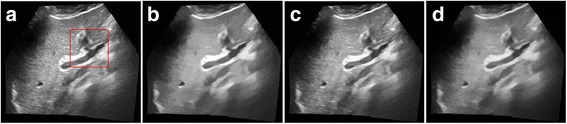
Results obtained with the compared filters applied to the 3D ultrasound image. a The original image. b-d Outputs of the compared filters, b BNLTV, c SAR-BM3D, d The proposed method. BNLTV = Bayesian NLTV; SAR-BM3D = blocking matching 3D filter of SAR image
